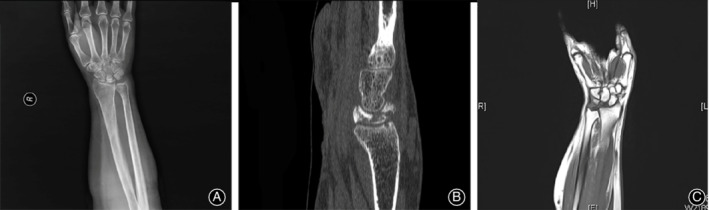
Preoperative radiographic views of the necrotic lunate with Lichtman classification IIIC. (A) X‐ray; (B) Computerized tomographic scanning; (C) Magnetic resonance image.
